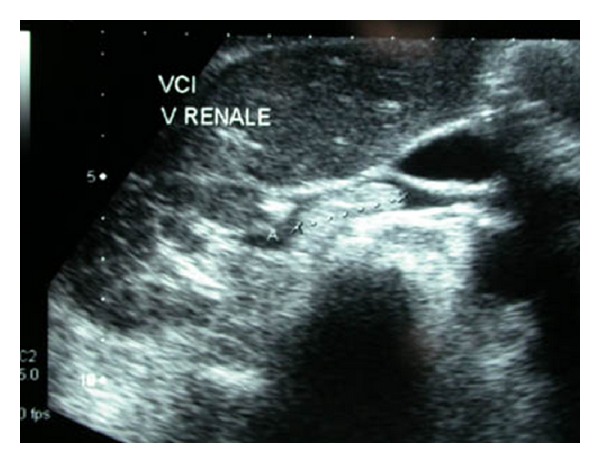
Sonography of the IVC thrombus originated from the right renal vein.
